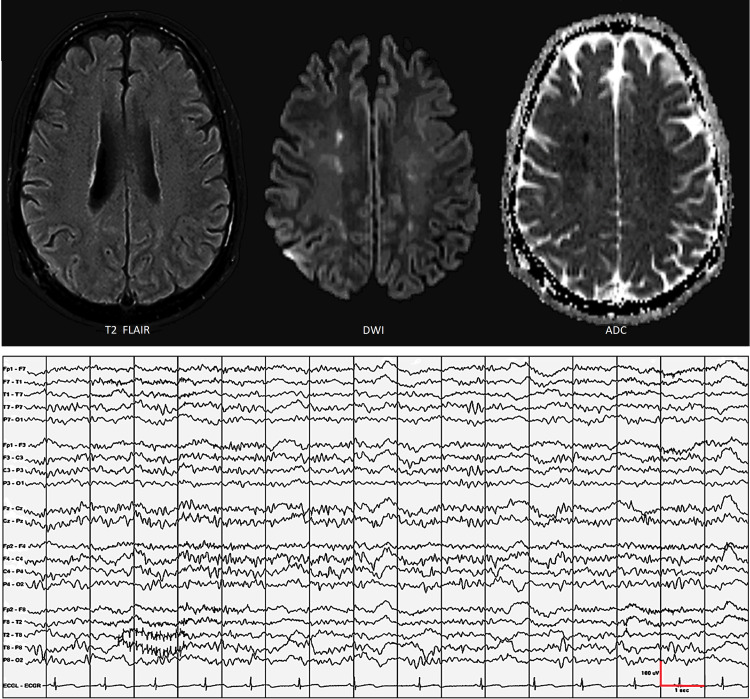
During initial admission because of acute opioid overdose, complicated by acute respiratory failure, hypotension, and obtundation, brain MRI showed watershed infarcts in the centrum semiovale and EEG showed diffuse theta-delta activity consistent with global hypoperfusion.
